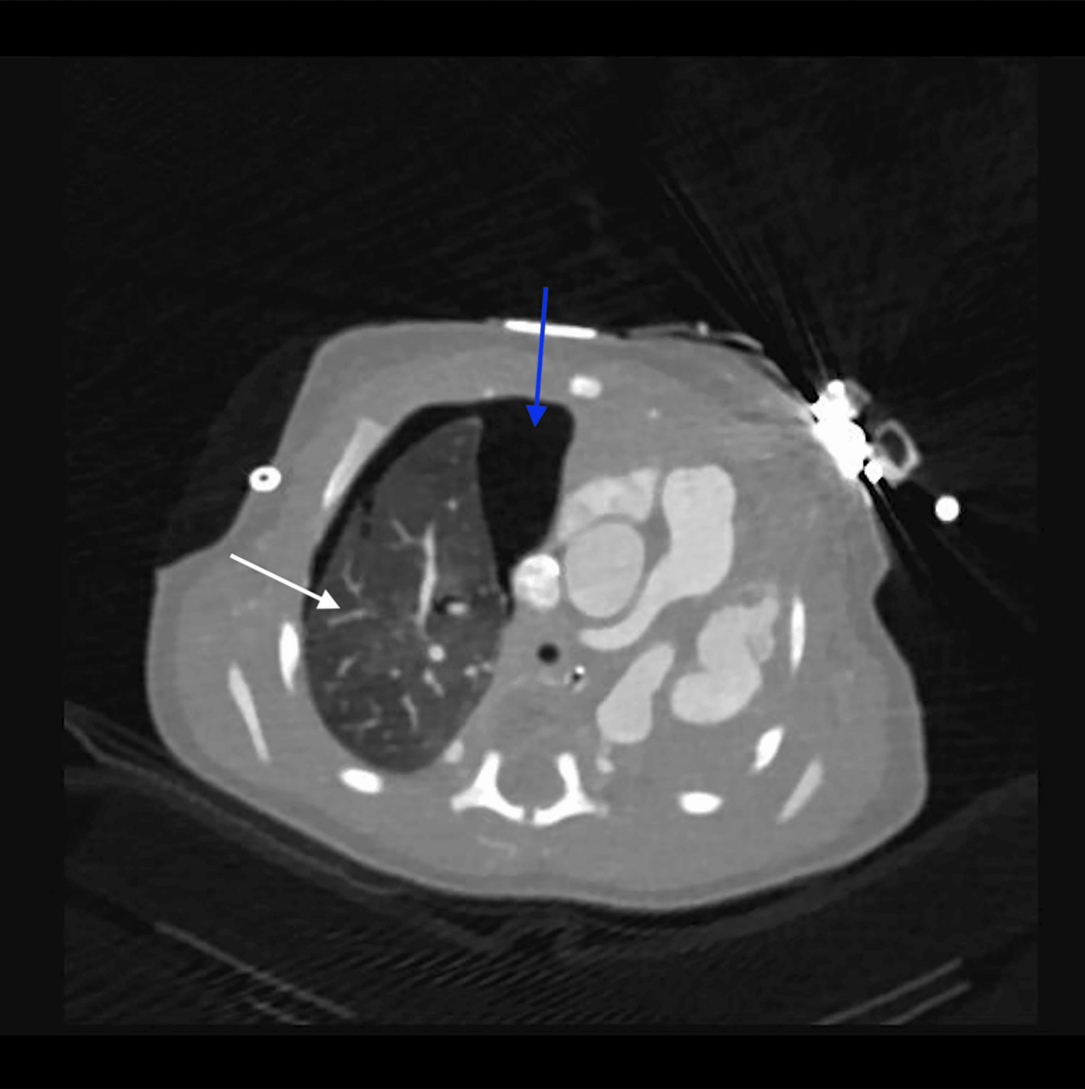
Axial computed tomography scan demonstrating complete absence of the left lung with a shift of the mediastinum to the left The right lung is indicated by the white arrow a right-sided pneumothorax is present (blue arrow).
